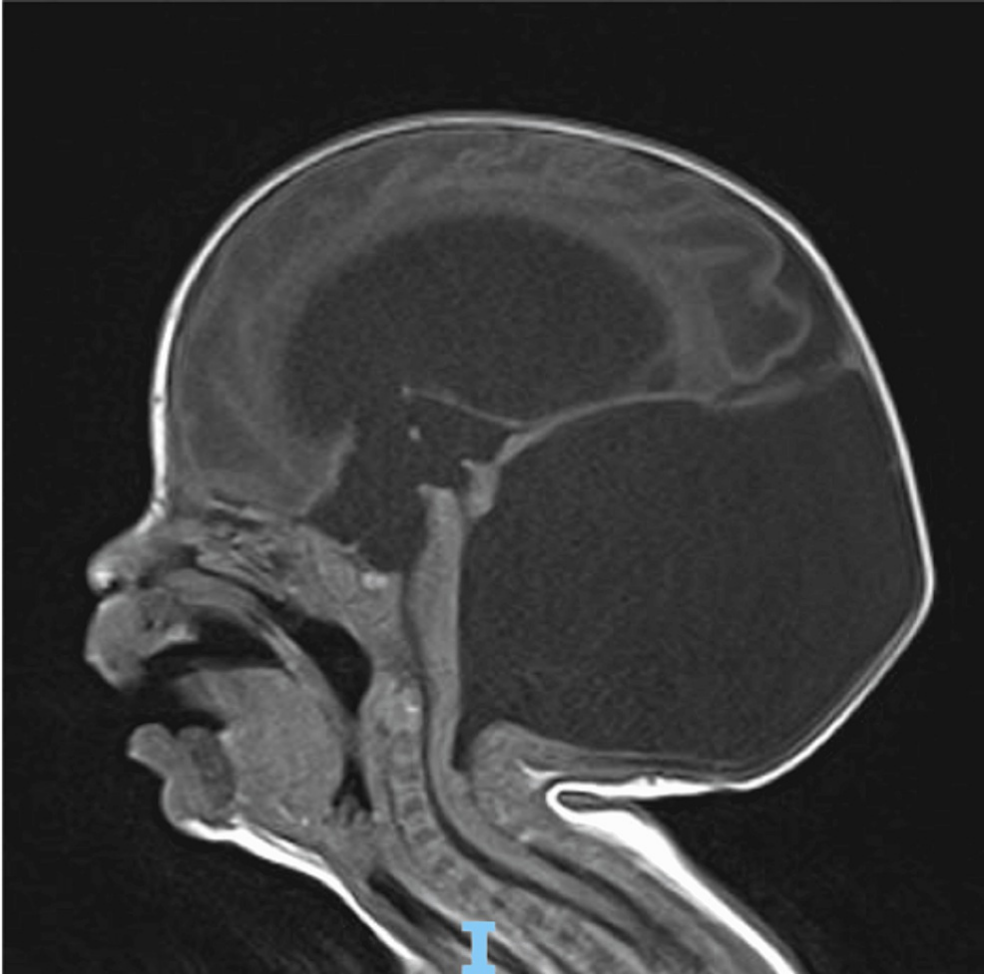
MRI of the brain showing marked hydrocephalus with a severely dilated fourth ventricle occupying the enlarged posterior fossa.
